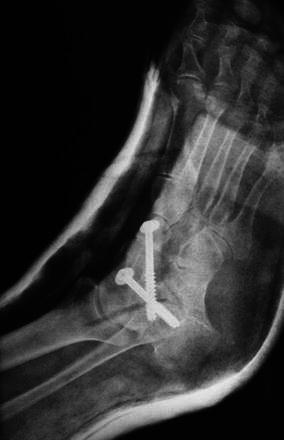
The X-ray shows the talus-scaphoid and talus-calcaneal arthrodesis and the two screws
